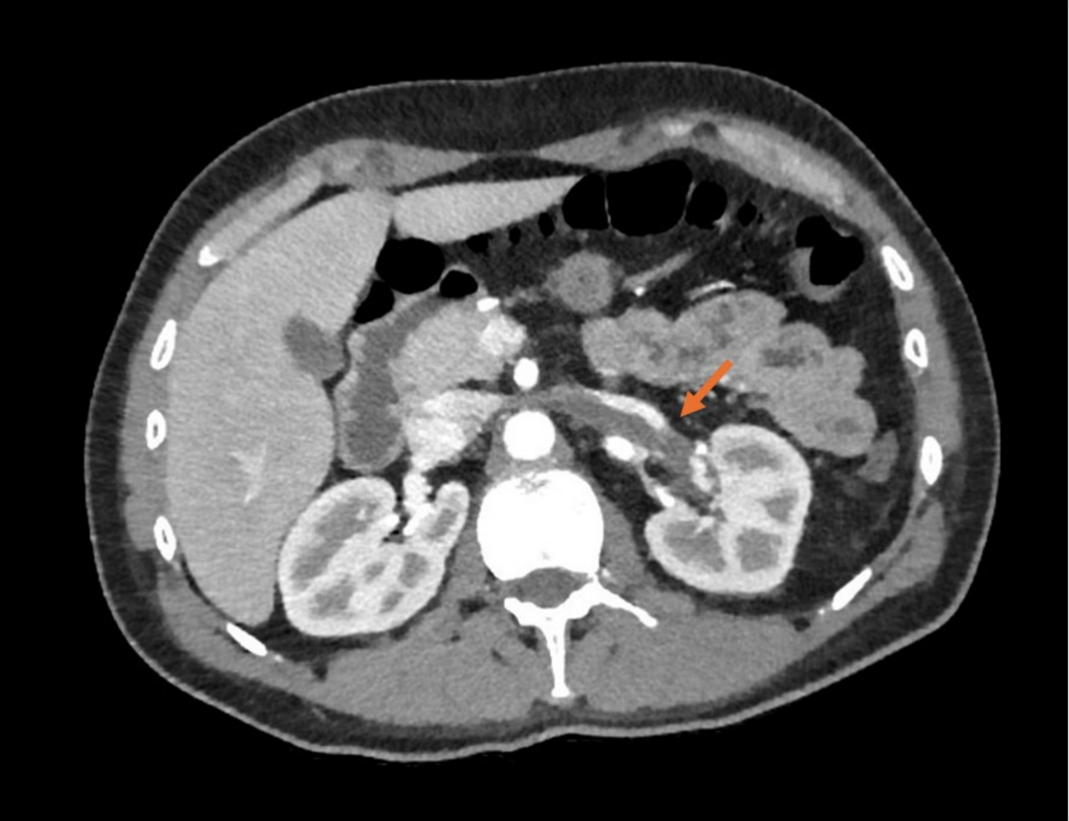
Thoracoabdominal CT angiography for detection of left renal vein thrombosis.
